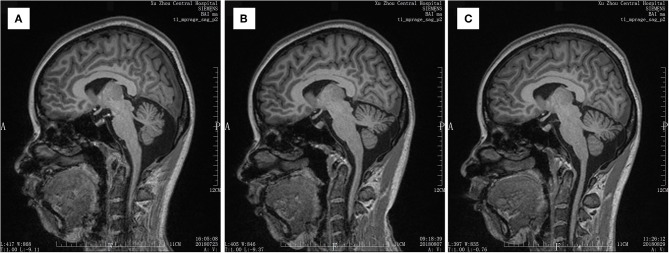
MRI T1 scan result of a patient. (A) Scan result before the surgery. (B) Scan result at 7 days after the surgery. (C) Scan result at 30 days after the surgery.
